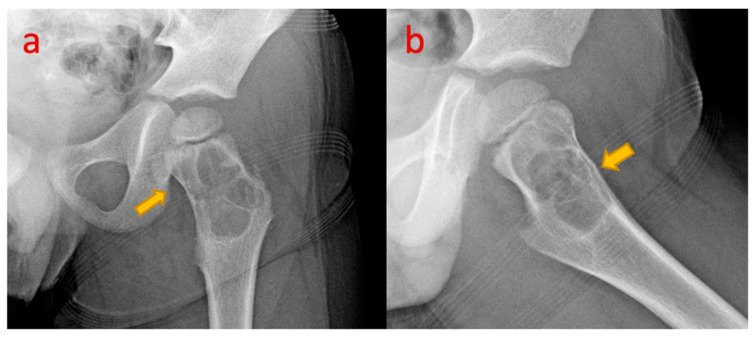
(a,b) Plain radiograph of the left hip in a 4-year-old boy. AP and frog leg lateral shows a pathological fracture of a bone cyst in the neck of the left femur. Anterior cortical break is appreciated on the lateral projection.
